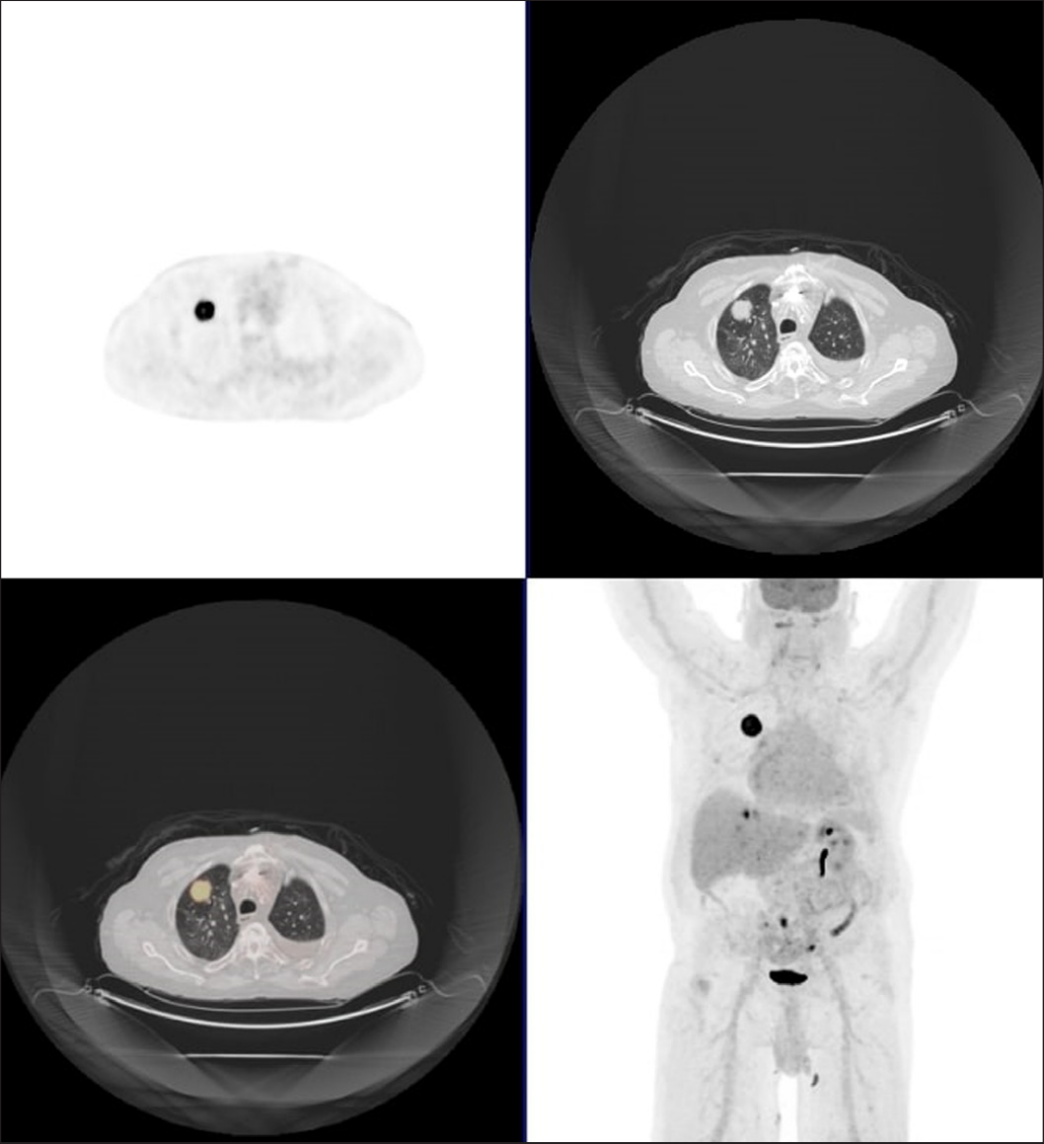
Fluorodeoxyglucose positron emission tomography SPECT imaging of demonstrating right upper lobe tumor (patient 2). SPECT: single photon emission computed tomography.
